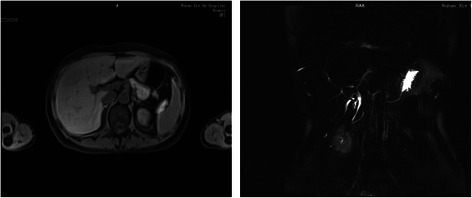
Enhanced Magnetic Resonance imaging(MRI) and Magnetic Resonance Cholangio Pancreatography (MRCP) of this patient were normal.
